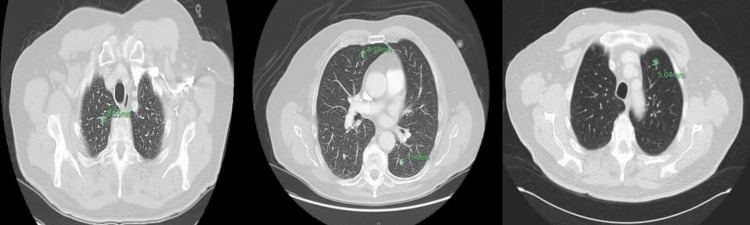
CT chest axial view one year after radiation therapy displayed the presence of six new pulmonary nodules.
